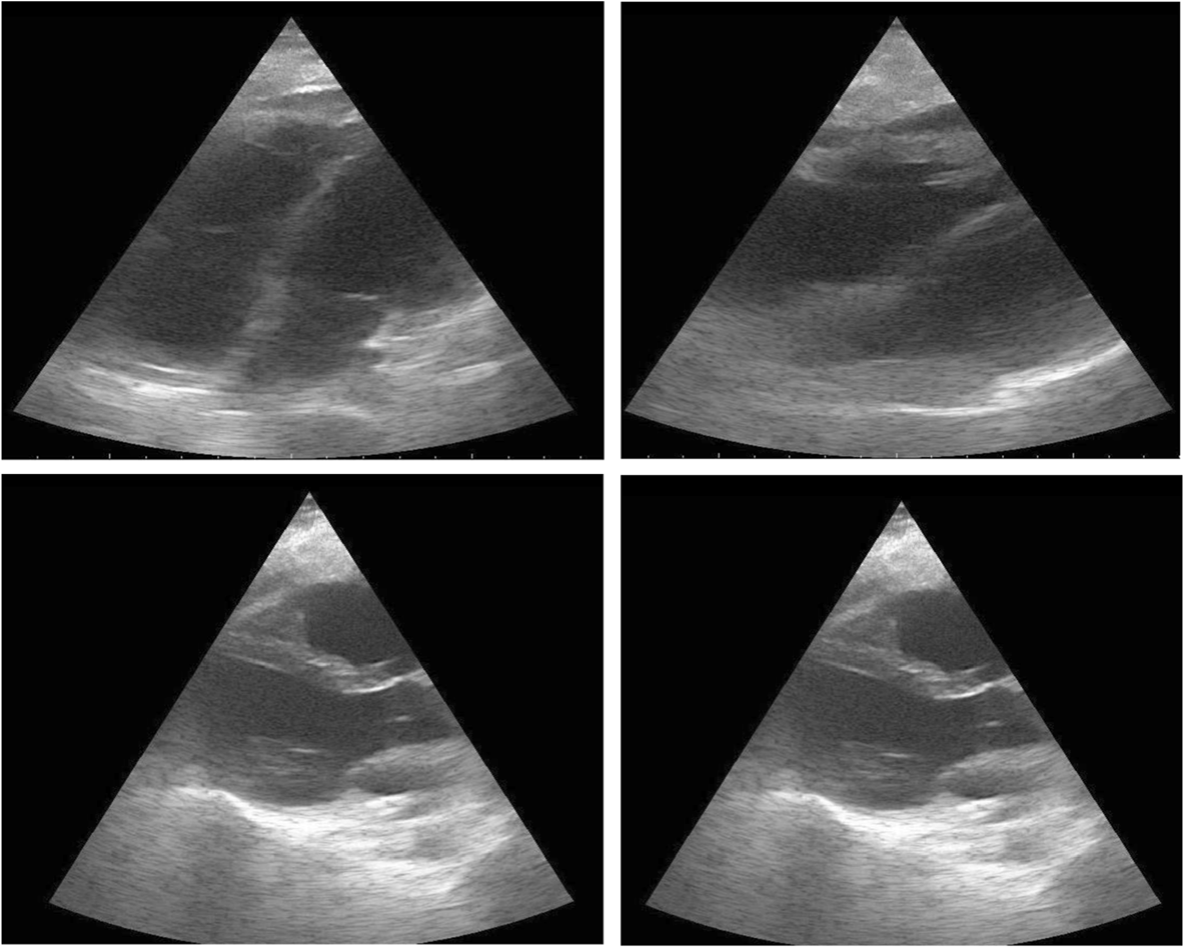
Echocardiogram. Showing areas of regional wall motion abnormalities
